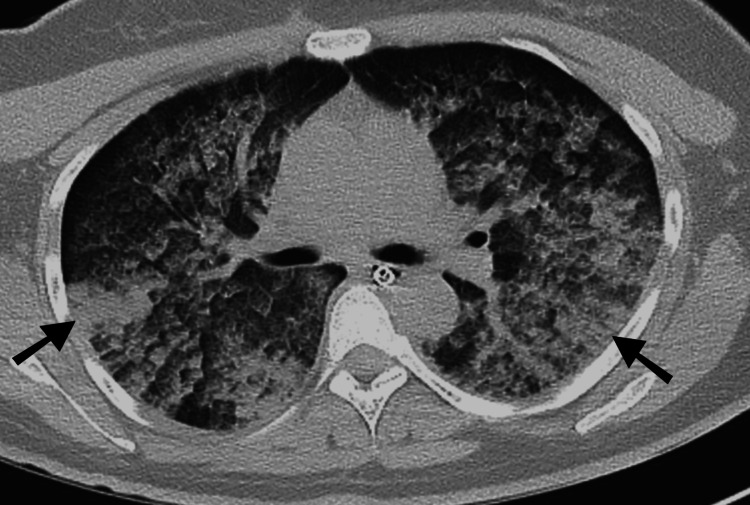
High-resolution CT (HRCT) chest done on day 4 showing ground-glass opacities in bilateral lung fields
